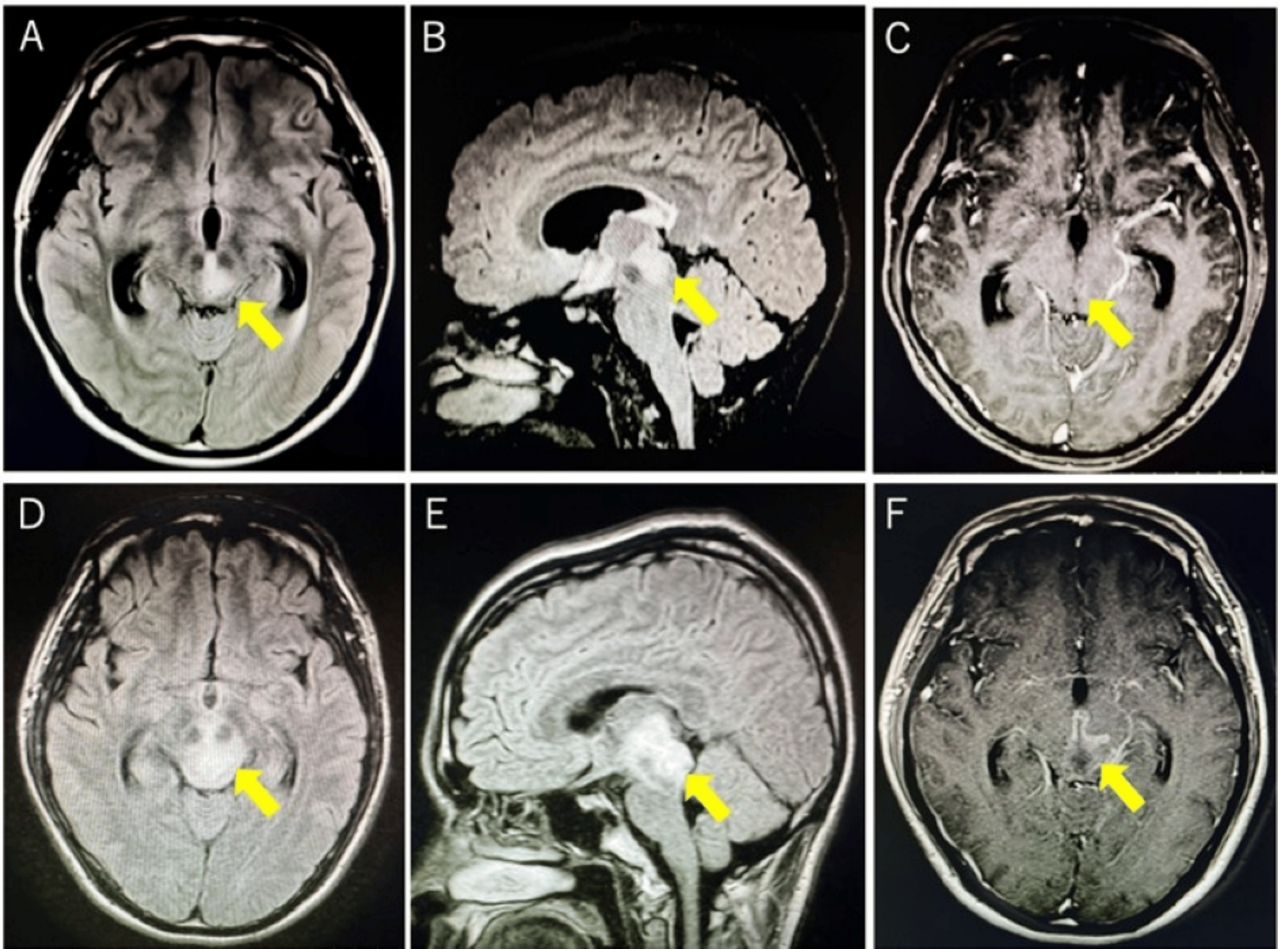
Brain MRI at initial presentation and postoperative day nine MRI findings at initial presentation (A-C). Axial (A) and sagittal (B) FLAIR images show a hyperintense lesion extending from the left thalamus to the left midbrain, accompanied by obstructive hydrocephalus with dilation of the third and lateral ventricles. (C) Axial T1-weighted postcontrast image reveals mild heterogeneous enhancement. Postoperative brain MRI findings on hospital day nine (D-F). Axial (D) and sagittal (E) FLAIR images show significant progression of the midbrain lesion, indicating increased inflammatory activity, whereas hydrocephalus has resolved. (F) Axial T1-weighted postcontrast image demonstrates enhancement of the lesion. MRI: magnetic resonance imaging; FLAIR: fluid-attenuated inversion recovery
